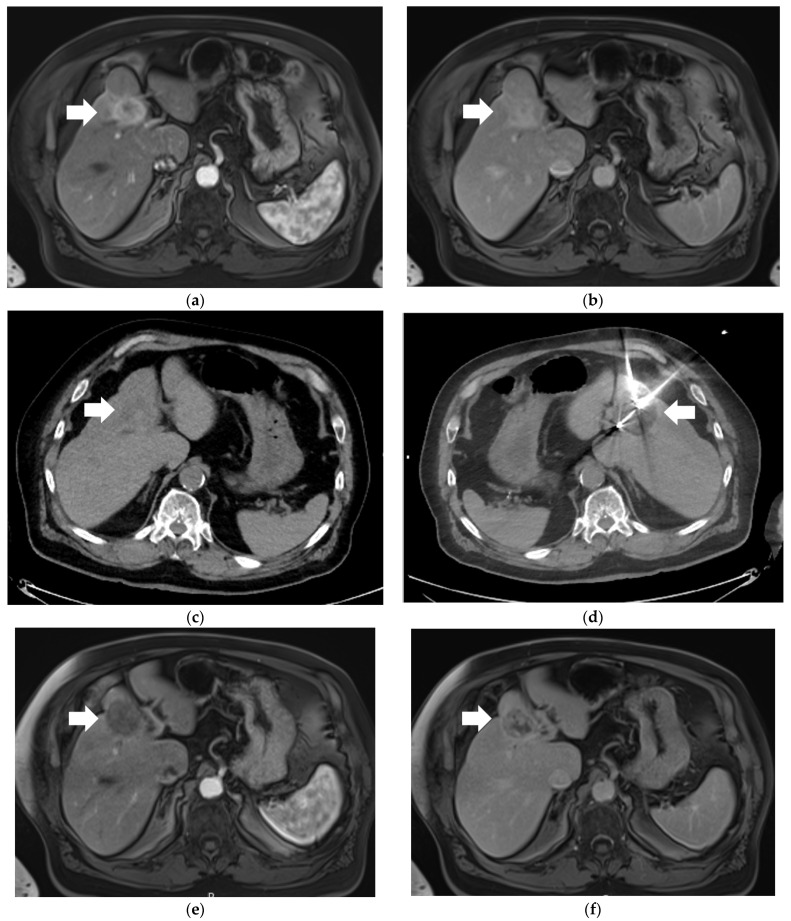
77-year-old male with a 3.7 cm intrahepatic cholangiocarcinoma (iCCA) in Segment 4A of the liver who underwent percutaneous cryoablation. (a) Selected arterial-phase contrast-enhanced T1-weighted magnetic resonance imaging (MRI) image demonstrating avid enhancement of the iCCA (arrow) compared to background liver parenchyma. (b) Selected delayed-phase contrast-enhanced T1-weighted MRI image demonstrating persistently elevated enhancement of the iCCA (arrow) relative to background liver. (c) Selected pre-procedural non-contrast axial CT image demonstrating the iCCA (arrow) as hypodense relative to background liver parenchyma. (d) Selected intra-procedural non-contrast axial CT image demonstrating two of a total of three cryoablation probes positioned within the iCCA, as well as associated “ice ball” (arrow). (e) Selected 1-month post-cryoablation arterial-phase contrast-enhanced T1-weighted MRI image demonstrating absence of previously seen tumoral enhancement. (f) Selected 1-month post-cryoablation delayed-phase contrast-enhanced T1-weighted MRI image demonstrating absence of previously seen tumoral enhancement.
